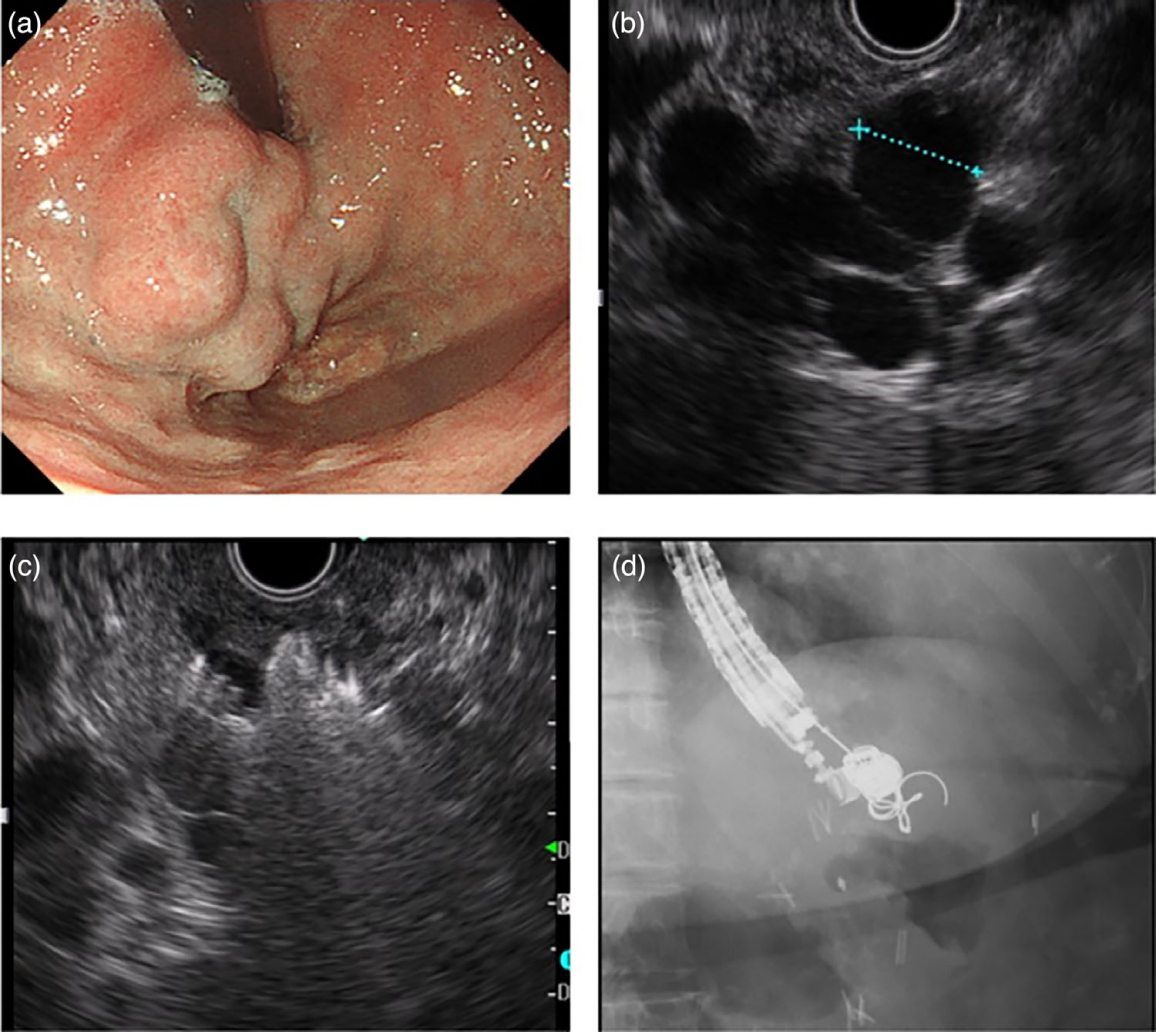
(a) Endoscopy showed Lg(f). (b) Endoscopic ultrasound (EUS) showed a varix diameter of about 12 mm. (c) Coil placement can be confirmed using EUS. (d) Fluoroscopic image after treatment. Fluoroscopy results confirmed that the coil is placed tightly.
